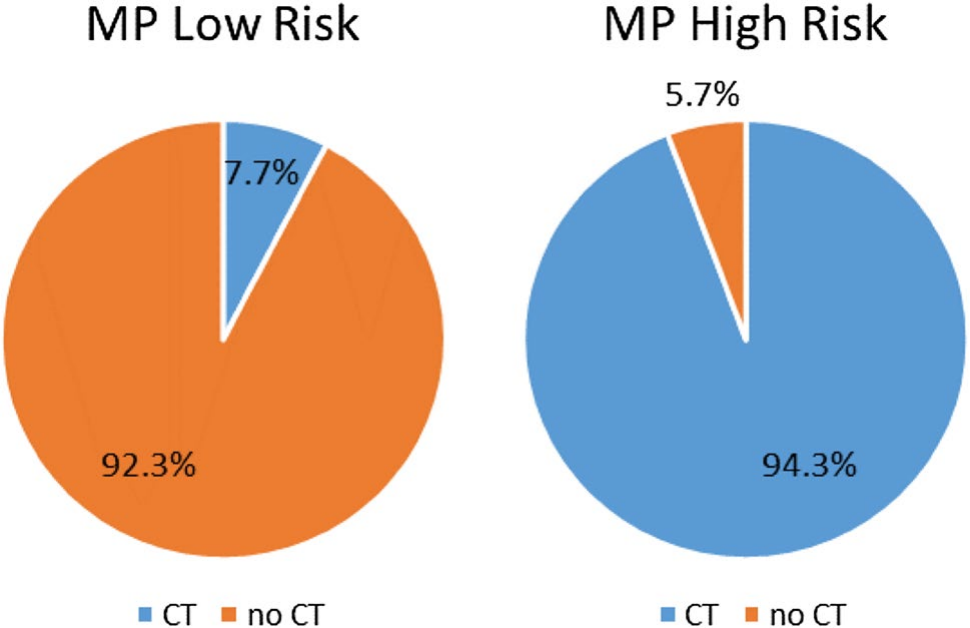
Percentage adherence to MammaPrint (MP) test result with respect to chemotherapy (CT) post-test chemotherapy recommendation
